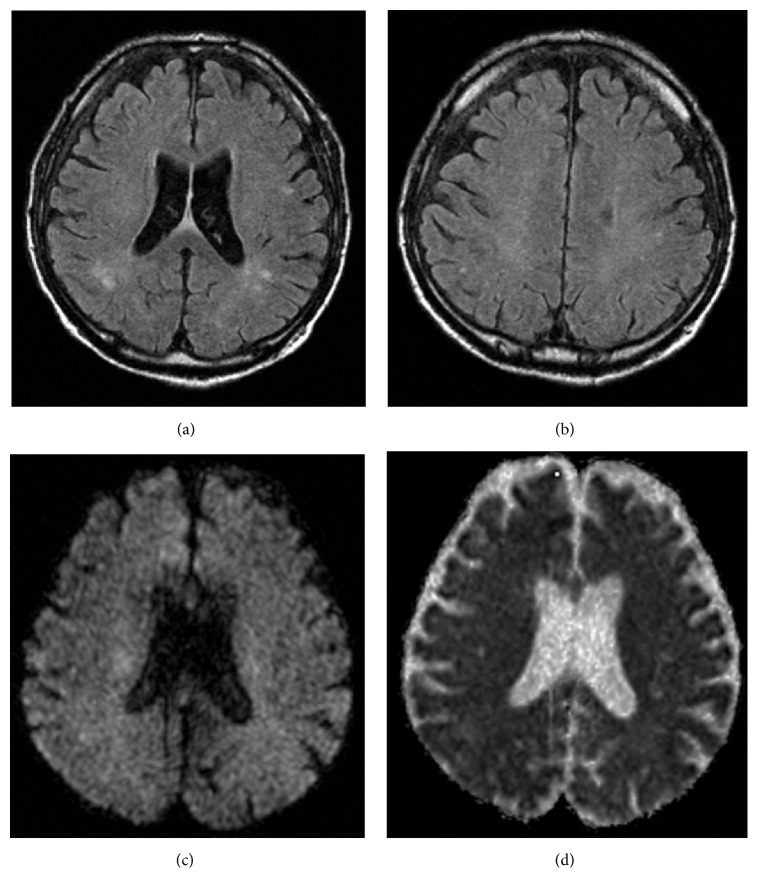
Cranial MRI on admission. ((a), (b)) Cranial T2-weighted fluid attenuated inversion recovery (FLAIR) MRI showing several old small brain infarctions. (c) Diffusion-weighted MR imaging (DWI) obtained on admission, showing no hyperintense signal alterations. (d) Apparent diffusion coefficient (ADC) mapping obtained on admission, showing no increased diffusion in cerebral lesions.
